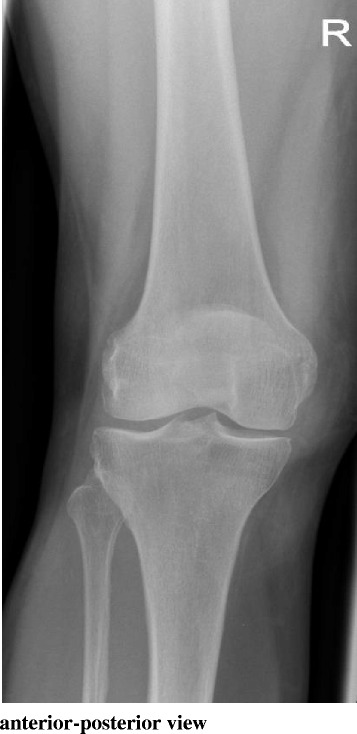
X-ray picture 4 months after surgery. Calcification deposit completely removed
